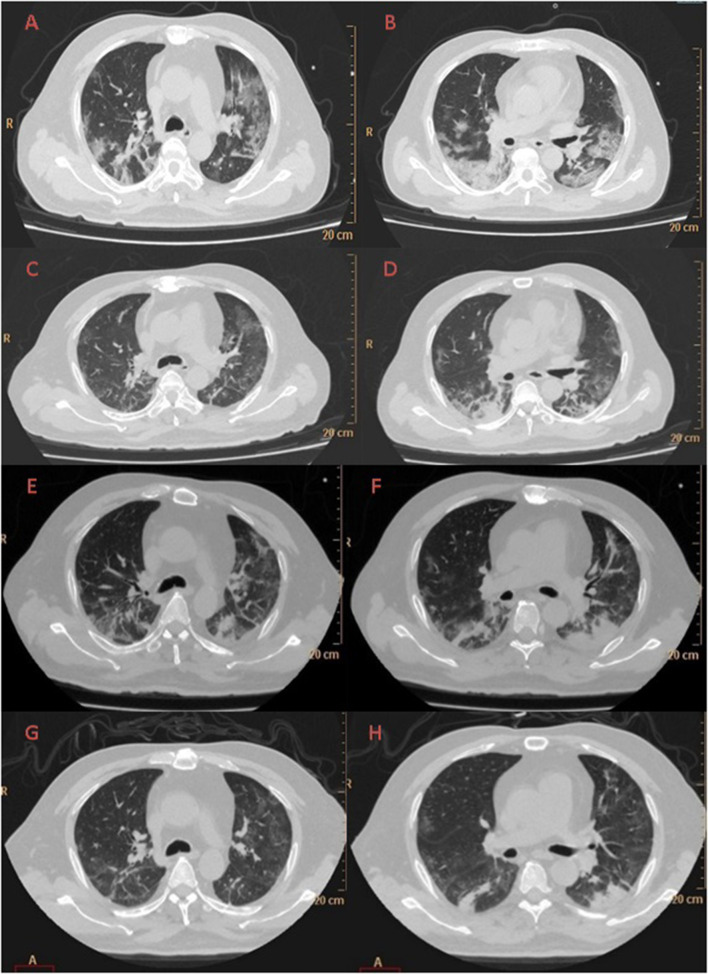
Evolution of the chest CT of the COVID-19 patient during hospitalization to ICU isolation ward of Liaocheng People-s Hospital: Day 1 (A,B) A large area of ground-glass opacity with uneven density was seen in the subpleural region of both lungs, with fine grid (crazy-paving sign), predominantly in the lower lobes. Multiple patchy consolidations were apparent in the lingular segment of the left upper lobe and bilateral lower lobes, with air bronchus-charging sign and thickening of the pulmonary interstitium surrounding the lesions. Day 6 (C,D) Patchy ground-glass opacity was seen in the subpleural region of both lungs, with multiple chords and consolidation shades in the bilateral lower lobes, but the extent decreased and the density thinned. Day 12 (E,F) There were reduced regions of initial ground-glass opacity, with new area of subpleural consolidation. Day 19 (G,H) Most of ground-glass opacity lightened or disappeared, partial area of consolidation was still observed.
